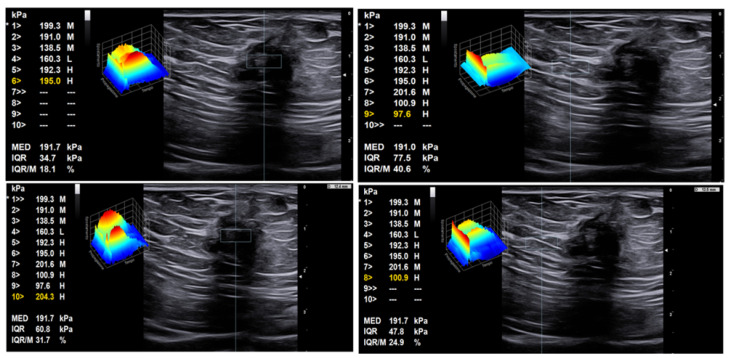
Share wave elastosonography mode revealing the hard pattern of the endometriotic nodule with respect to the surrounding tissue. Left: ROI within the tumor, with high shear wave pressures between 195 and 204 kPa; right: ROI within soft tissue surrounding the tumor, with distinctly lower shear wave pressures of 98–101 kPa.
